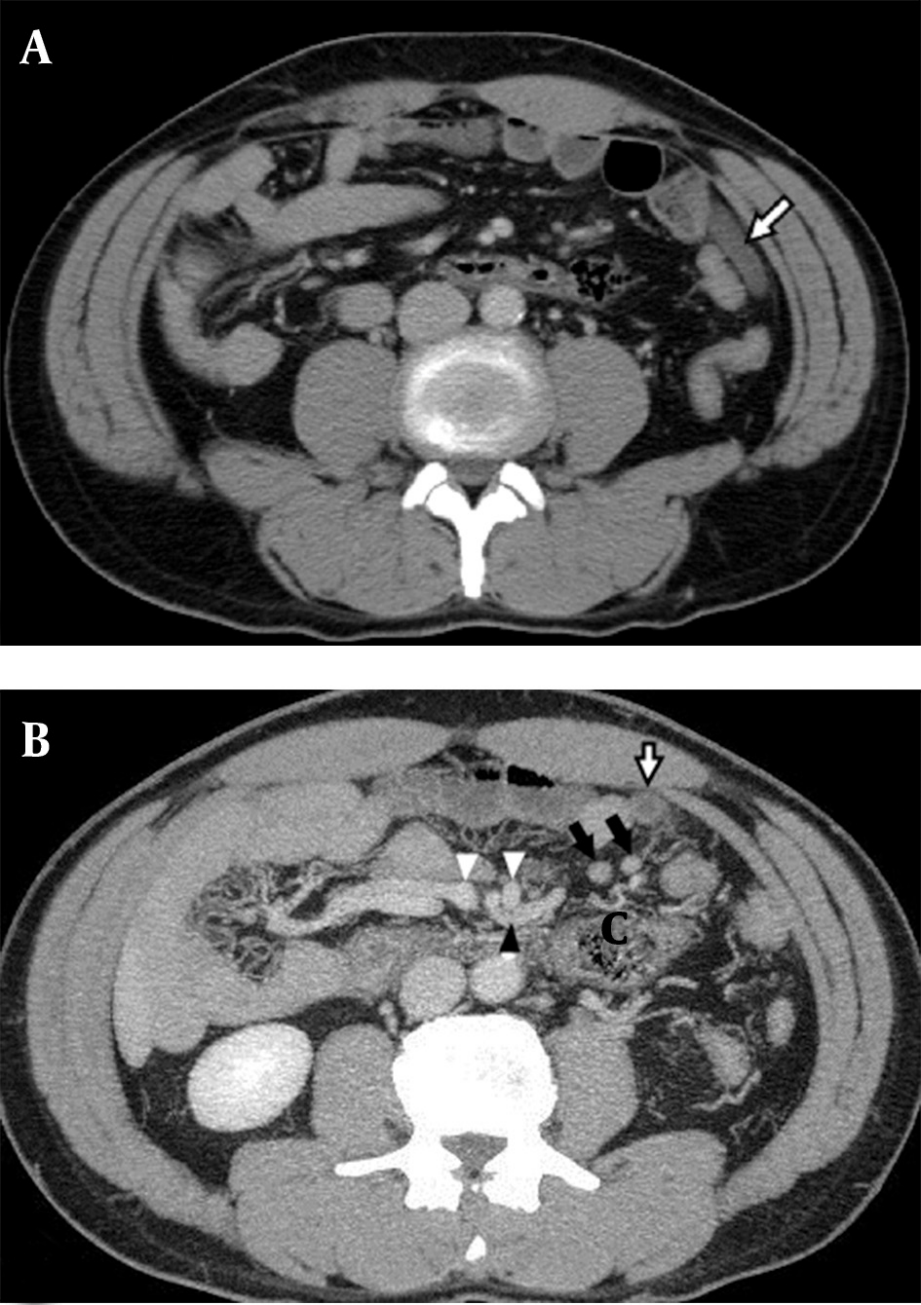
A, The CT image shows a misplaced ascending colon with the cecal base located in the LUQ of the abdomen. Swelling of the appendix (white arrow) (0.8-cm calibre) is seen. B, The maximum intensity projection of axial plane with 20 mm thickness shows swelling of the appendix (white arrow) in the anterior aspect of the cecum (C), with few inflammatory and enlarged lymph nodes (black arrows). The ileocolic artery (black arrow head) arises from the superior mesenteric artery, between the inferior mesenteric vein (right white arrow head) and the superior mesenteric vein (left white arrow head). The ileocolic artery is passing under the inferior mesenteric vein and supplies the cecum in the LUQ of the abdomen.
